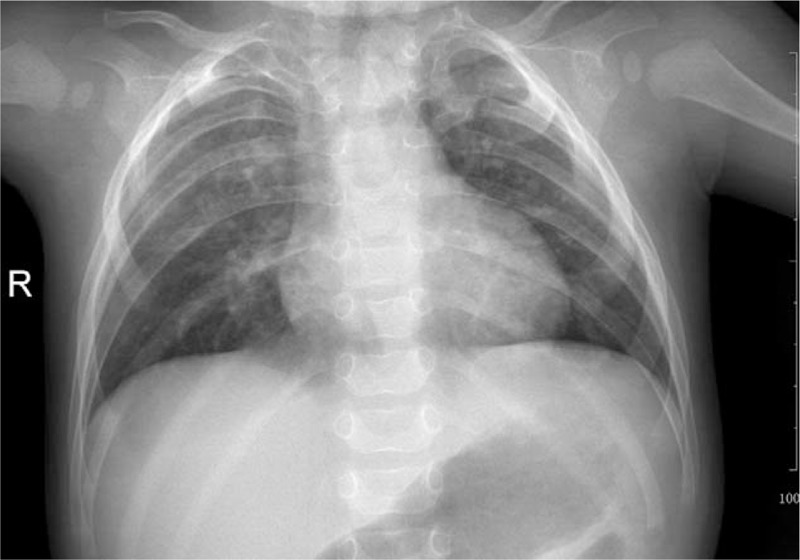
Anterioposterior chest radiograph of the 10-month-old boy with the VACTERL association. The radiograph showed patchy shadows, thoracic vertebral anomalies and rib anomalies (only 10 sets of ribs were seen. The posterior part of the upper ribs was with an irregular shape and the right 2nd, 3rd ribs and the left 2nd rib were short. The upper middle part of the thoracic vertebra was not shown clearly).
